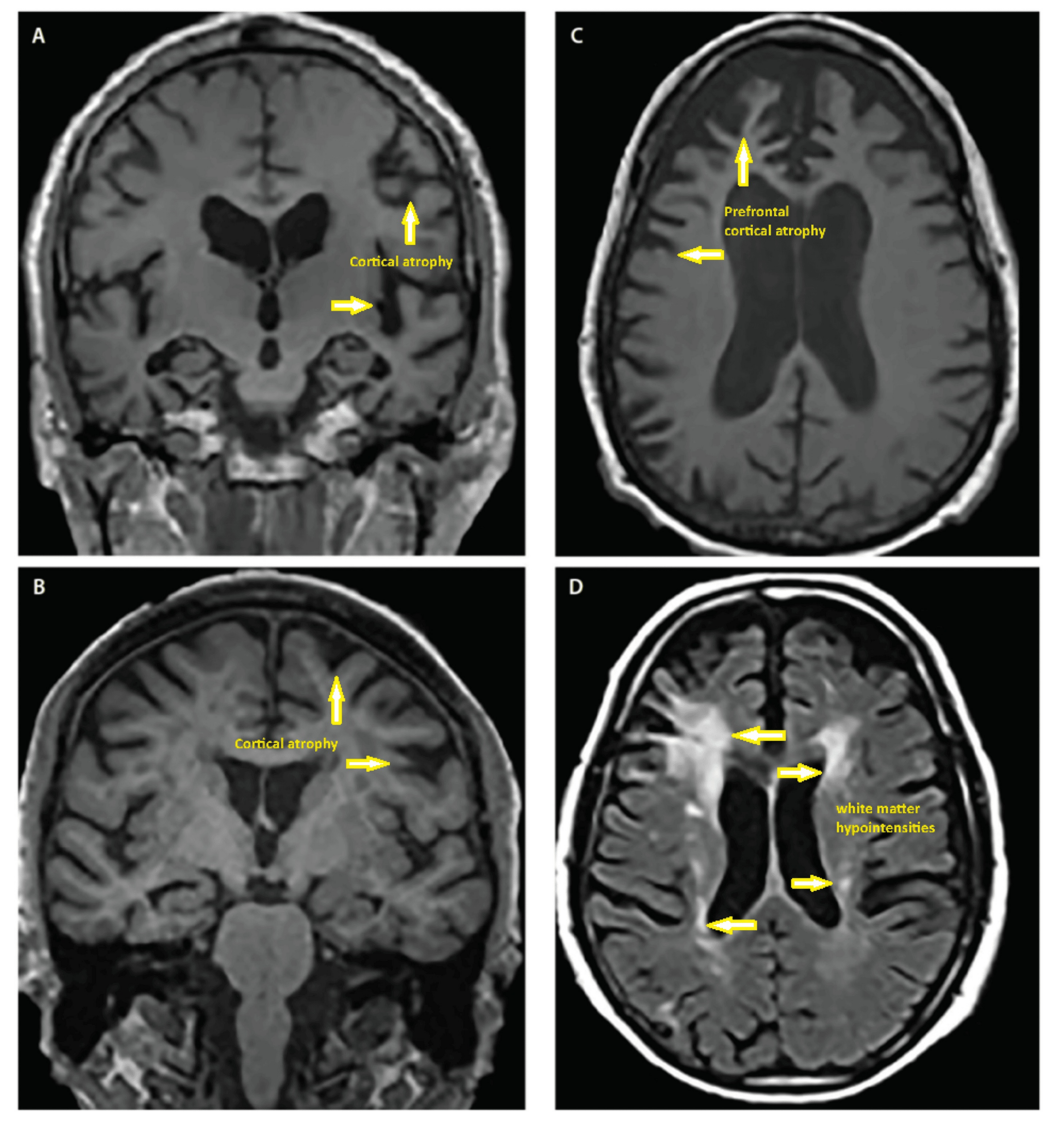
MRI images of dementia. Structural atrophy patterns across Alzheimer’s disease (AD) (A), dementia with Lewy bodies (DLB) (B), frontotemporal dementia (FTD) (C), and vascular dementia (VaD) (D). Coronal T1-weighted MRI demonstrates generalized cortical atrophy in AD (A) and DLB (B). Axial T1-weighted MRI demonstrates biventricular frontal and temporal atrophy with a predilection for the medial and lateral prefrontal cortices (C). Axial T2-weighted MRI demonstrates confluent periventricular cap and halo white matter hypointensities involving the corona radiata, lateral prefrontal neocortex, subependymal ventricular lining and septum pellucidum in VaD (D) [13].
